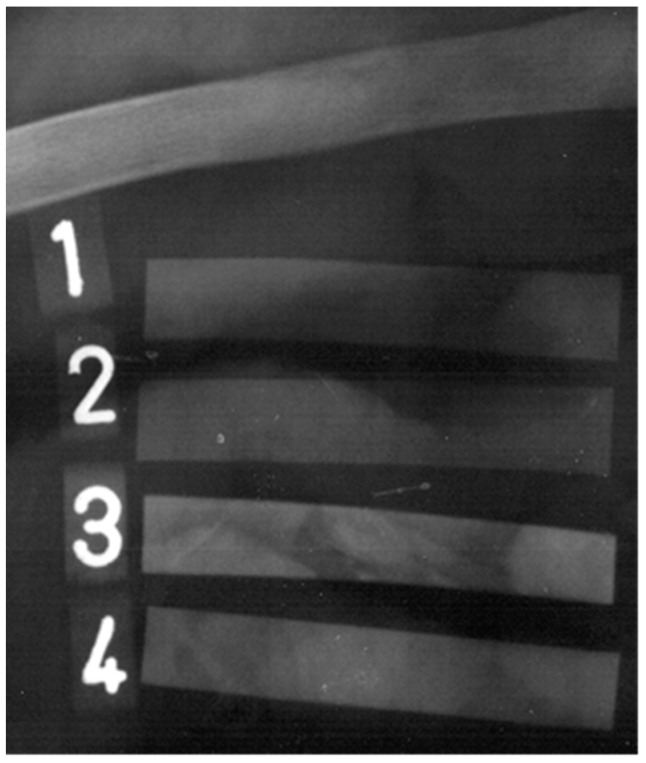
X-ray image of the radiopaque samples. The numbers 1, 2, 3, and 4 stand for the samples 10Bi, 17Ba, 20Bi, and 34Ba, respectively. In contrast, a bone is presented in the top part (above sample 10Bi).
